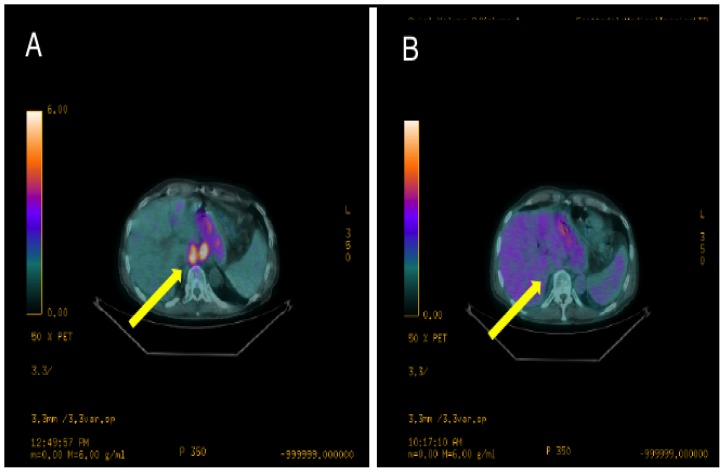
Patient 9 PET/CT images. 18-fluorodeoxyglucose positron emission tomography/computed tomography (PET/CT) images depicting (A) axial slice at baseline and (B) axial slice after 30 days on treatment. The yellow arrow is pointing to two hypermetabolic lymph nodes in B and C. In B, the standard uptake value (SUV) of the left lymph node is 9.8 and the lymph node on the right is 7.5, while the SUV of the left lymph node is 3.1 and the lymph node on the right is 3.5 in C.
